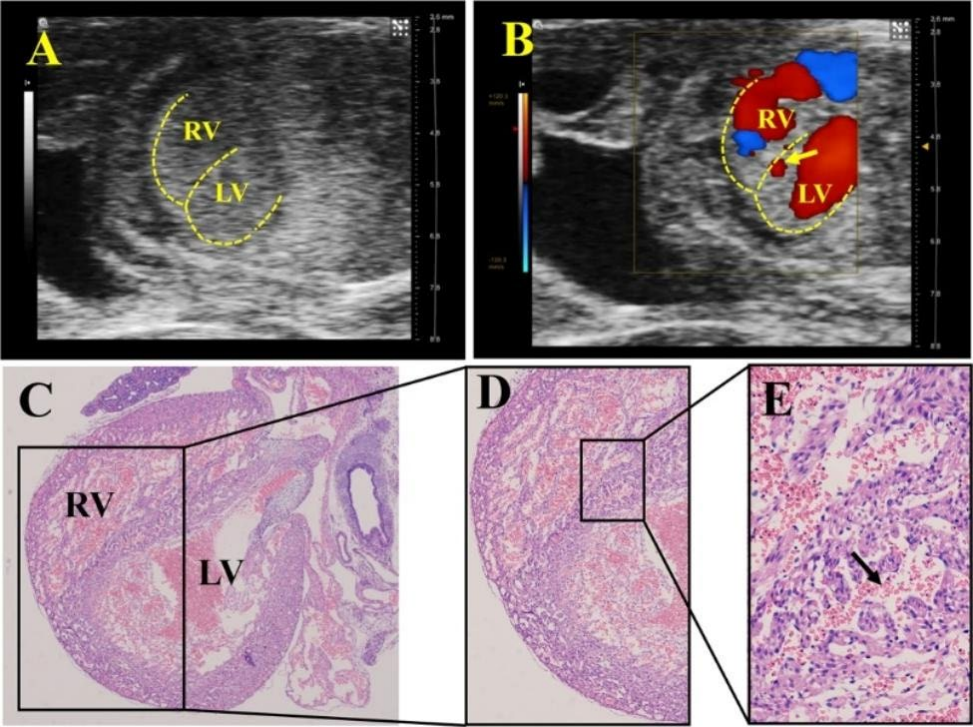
Prenatal ultrasound and pathological images of fetuses with a “spongy” myocardium. A is a B-mode image; B is a color Doppler image, revealing that a small amount of blood flow signals appears in the septum (arrow). C, D, and E are all pathological images (magnifications are 40×, 100×, and 400×, respectively). The ventricular septum was roughly intact, but the myocardium was loosely arranged with RBCs crossover (arrow). (The dotted line indicates the ventricular wall and septum. LV, left ventricle; RV, right ventricle)
